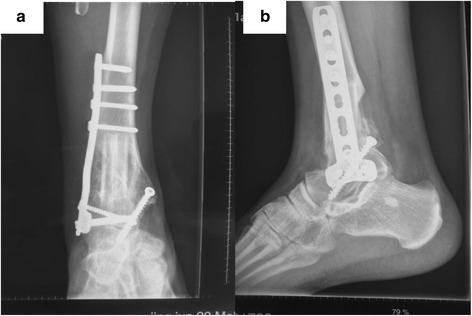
X-ray, 28 months after operation, shows the hypertrophy of the fibula and union of the junctions of the tibia-fibula and the fibula-talus. a AP X-ray. b lateral X-ray
